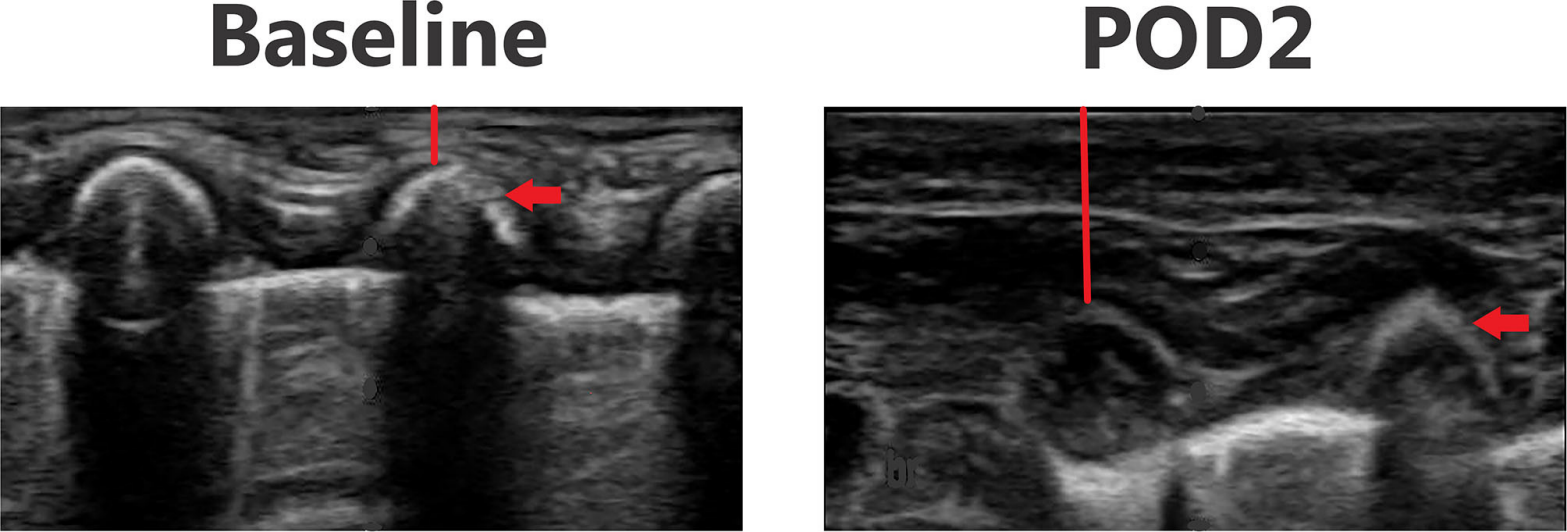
Point-of-care ultrasound images of lateral chest at baseline and POD 2 of the same patient. Both images are presented on identical scales (total depth of 1.5 cm from the skin). Red vertical line demonstrates subcutaneous tissue thickness—there is a marked increase in subcutaneous tissue seen on POD 2. Red arrow—rib bone.
